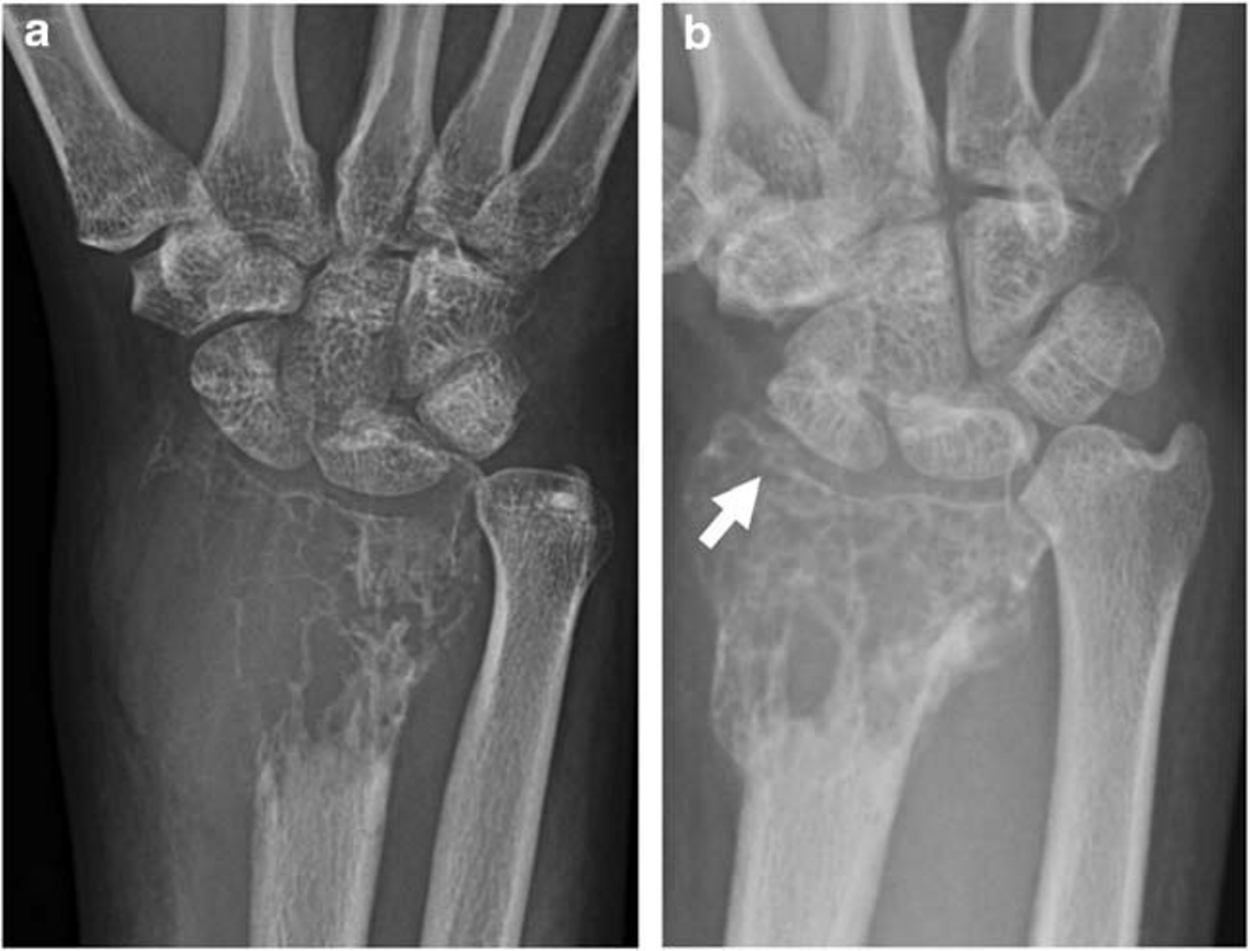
A 38-year-old female presented with wrist pain and swelling. Plain radiographs at presentation (a) and after 4 weeks on denosumab (b). a At presentation, a Campanacci grade 3 expansile lytic GCTB was found in the distal radius meta-epiphysis, extending up to the subchondral bone plate with destruction of the lateral radial cortex and radial styloid articular surface. b After 4 weeks of denosumab treatment, the cortex has reconstituted laterally with remodelling and irregularity of the distal radial articular surface (arrow). The soft tissue component of the lesion has markedly decreased in size. The distal radius shows an increase in matrix osteosclerosis
